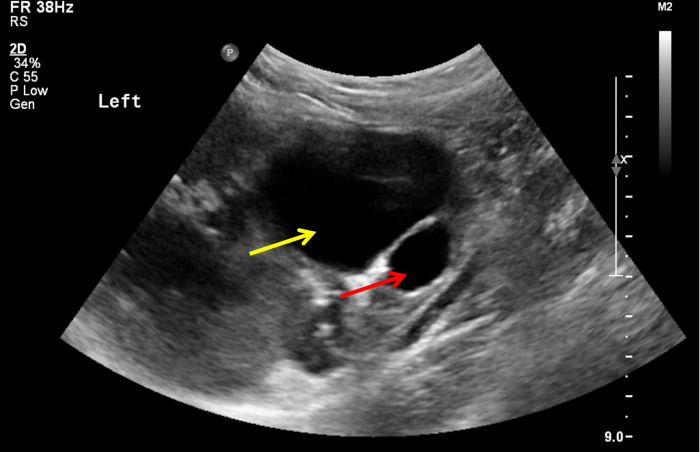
Image of ultrasound: unicystic tumor (yellow arrow) in the left kidney with a clear boundary and uniform internal sound transmission, accompanied by a dilatation of the renal pelvis (red arrow).
